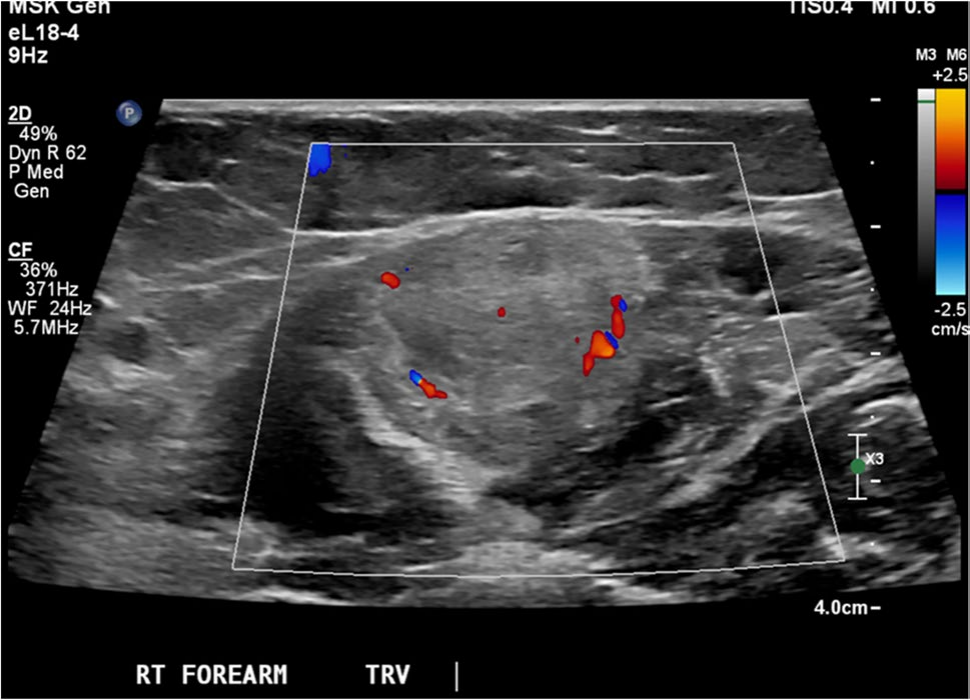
Ultrasound images demonstrate a heterogenous oval mass that is hyperechoic to adjacent musculature. There is internal color doppler flow
